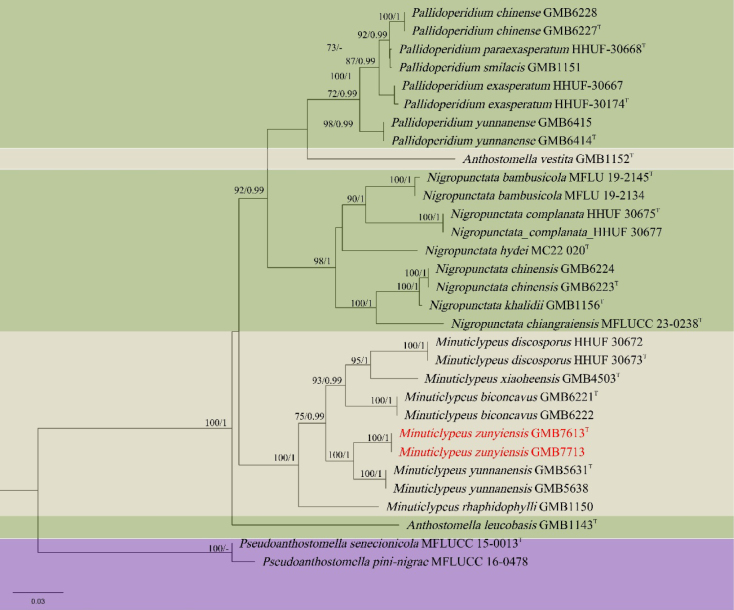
RAxMLtree of *Minuticlypeus* species and related taxa based on combined ITS, LSU, *tub2*, and *rpb2* sequences. Bootstrap support values for maximum likelihood (ML) greater than 70% and Bayesian posterior probabilities (BPP) greater than 0.90 are displayed above the respective branches (ML/BI). The best-scoring RAxML tree showed a final maximum likelihood optimization value of −12274.915712. Estimated base frequencies were as follows: A = 0.247642, C = 0.245222, G = 0.265487, and T = 0.241649. Substitution rates were AC = 1.153534, AG = 3.174185, AT = 1.383242, CG = 0.820682, CT = 6.338643, and GT = 1.000000. The gamma distribution shape parameter alpha was 0.884372, and the tree length was 1.126155. Type materials are marked with “T”, and the strains of newly described species are highlighted in red.
